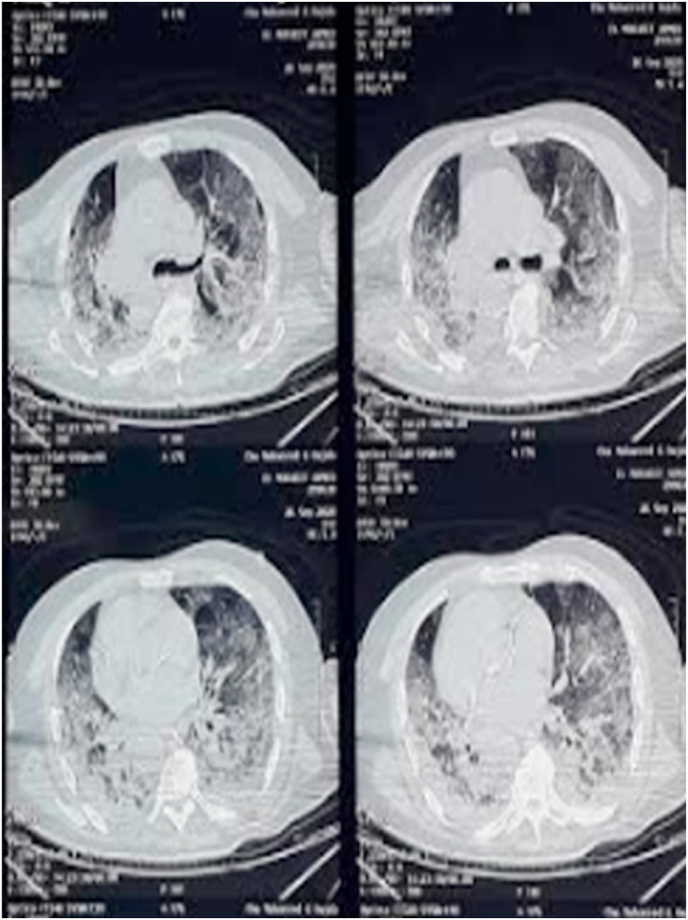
Pre-operative computed tomography scan of an 80 years old man with COVID-19- related pneumonia and acute limb ischemia. Transverse thin section scans show extensive ground-glass opacities of both lungs.
